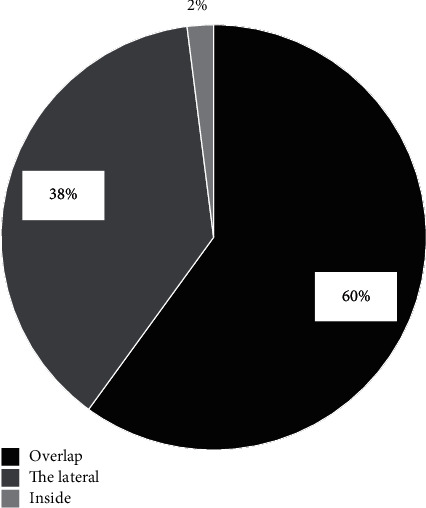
The proportion of the nerve location on the body surface in the scapula region of the traditional method and that under ultrasound.
